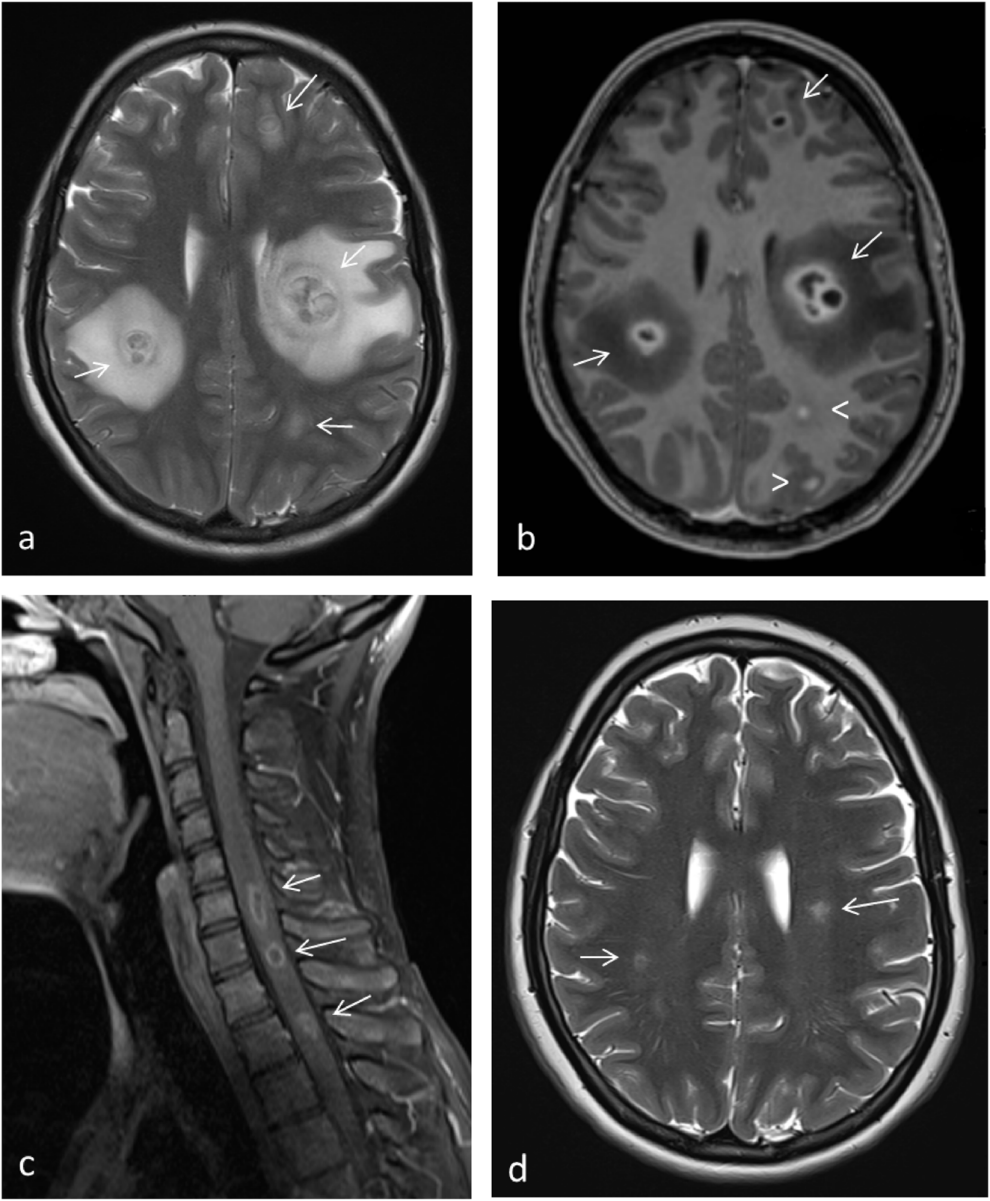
a–d: MRI T2 weighted imaging revealed multiple lesions with perifocal oedema (arrows) (a). Most of the lesions had ring-enhancement with gadolinium compatible with abscess (arrows). There were also nodular enhancing lesions (arrowheads) (b). Spinal cord MRI shows multiple ring-enhancing lesions (arrows) (c). The latest control image shows only small residual T2-lesions (arrows) that had remained stable over 8 months after completion of 35 months of voriconazole treatment (d).
